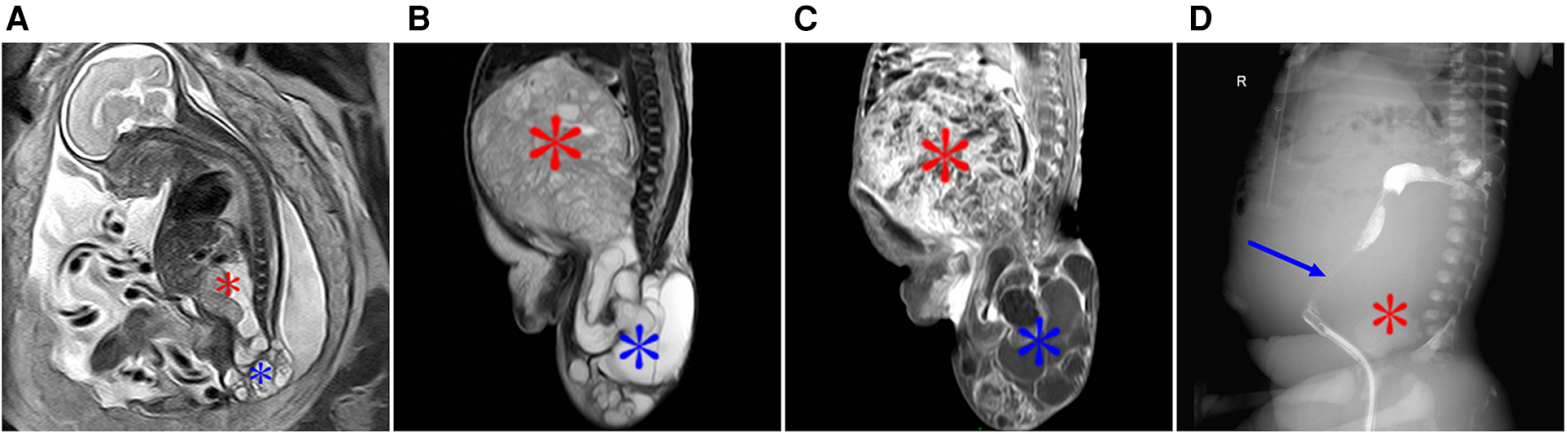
A type II sacrococcygeal teratoma at 25 weeks of gestation. (A) Mixed signals occupying both the front and the lower part of the sacrococcygeal region of the fetus. The lesions in the front of the sacrococcygeal region were mainly soft-tissue signals (red star), while the lesions in the lower part of the sacral tail mainly showed multiple cystic components (blue star). (B) After birth, the anterior sacral caudal mass on T2-weighted imaging showed iso-hyperintensity (red star), and the lesions below the sacral caudal mass were mainly cystic (blue star) and were significantly larger than those in the fetal period. (C) After enhanced scanning, the solid components of the lesion showed significant enhancement, and the cystic partial wall showed linear enhancement. (D) Lipiodol enema demonstrates a compressed anteriorly displaced rectum.
